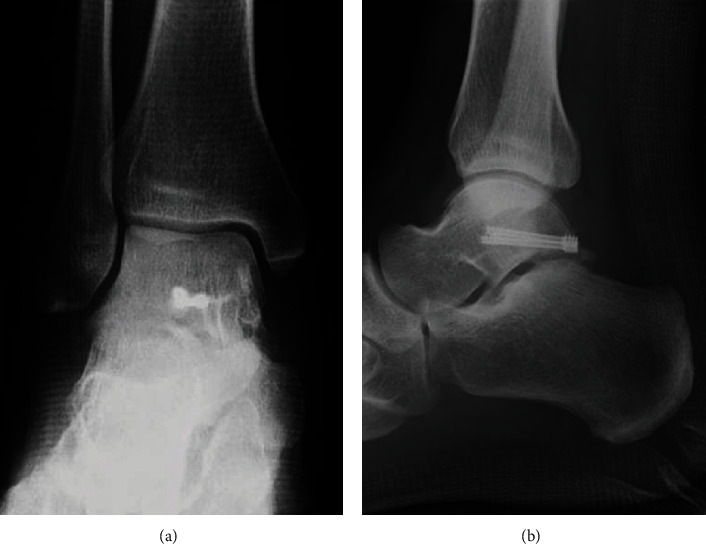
Postoperative plain radiograph in (a) anteroposterior and (b) lateral views showed that the fragment was reduced and fixed with two cannulated double-threaded screws.
